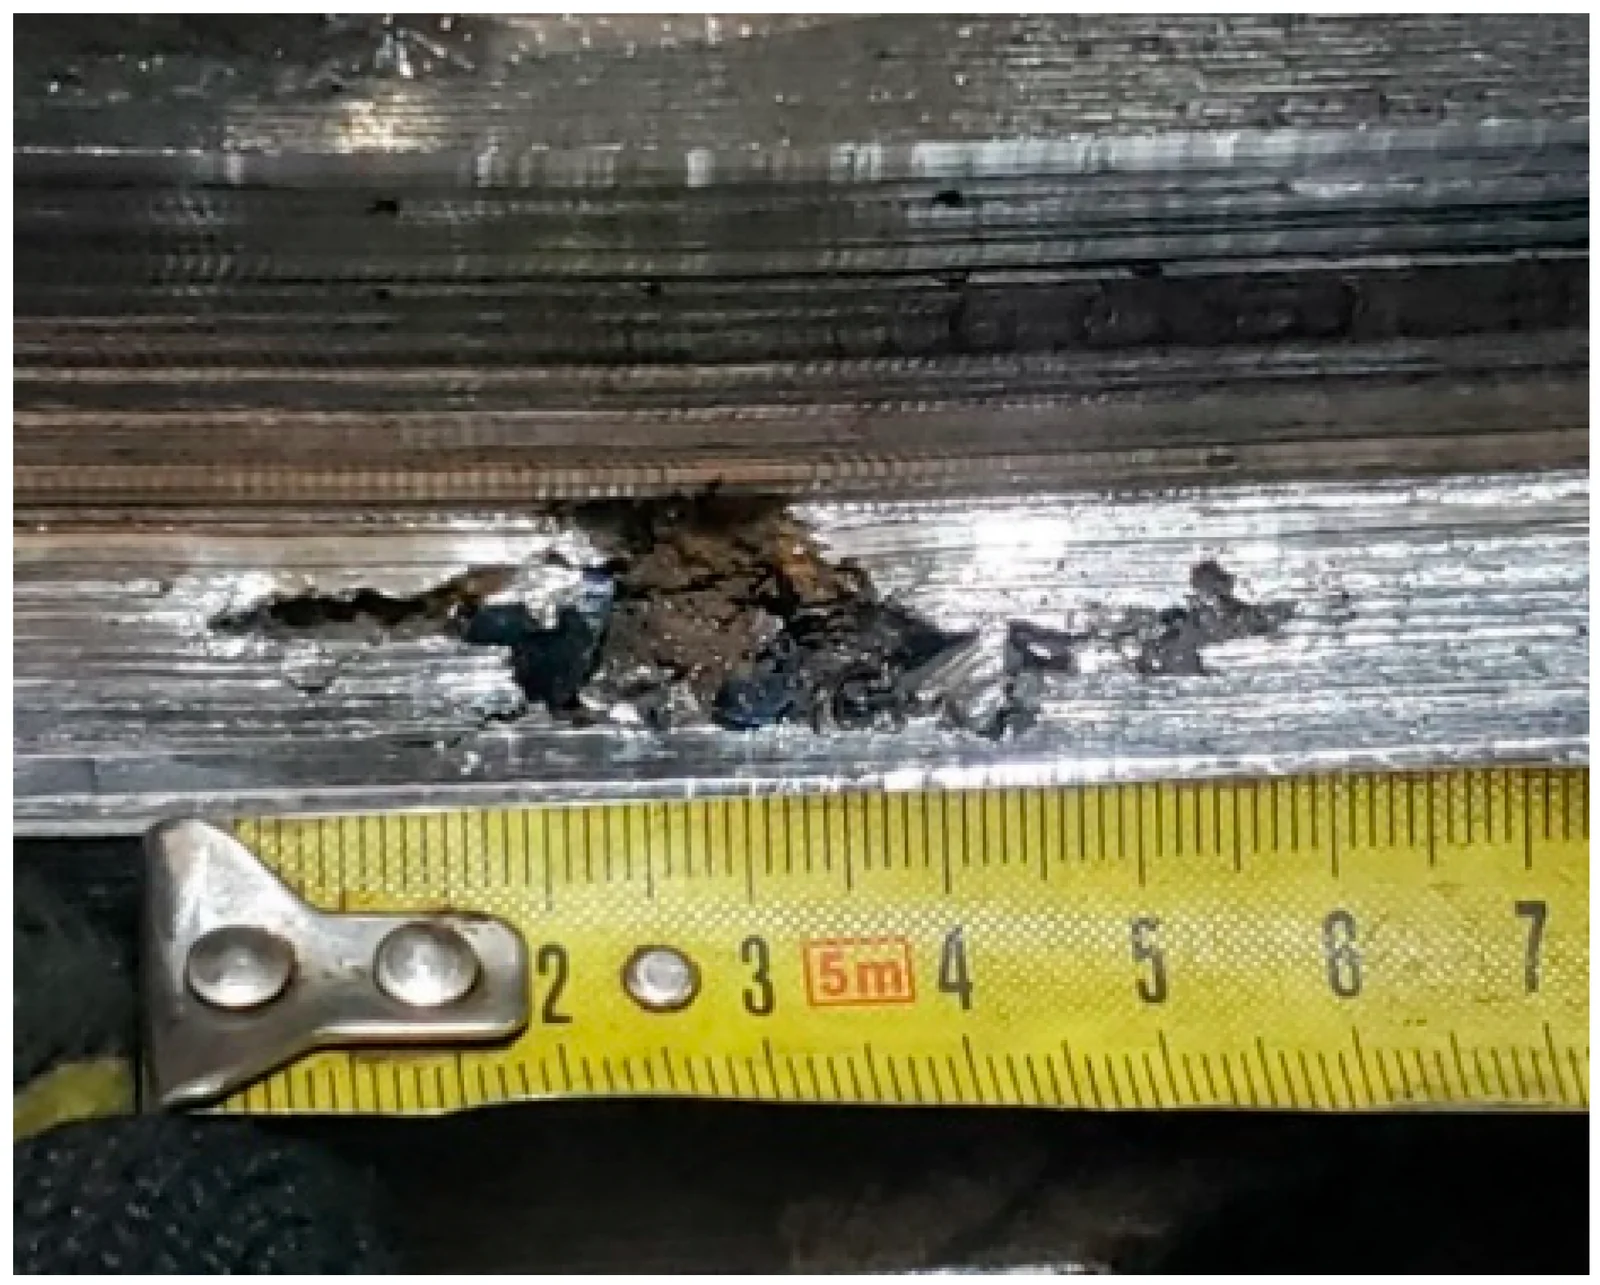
Close-up of a local cavity cluster exposed after sectioning the ultrasonically indicated area.
